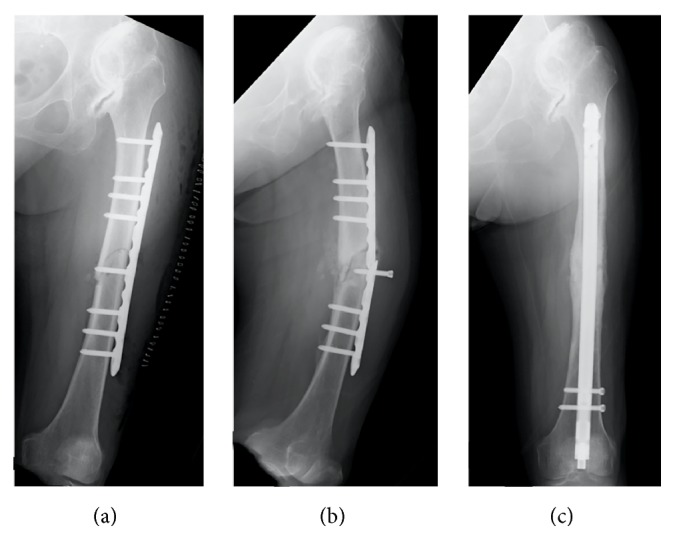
(a) AP image is shown, which was obtained after open reduction and internal fixation with an LCP plate. (b) AP radiographs obtained 22 weeks after fracture surgery show plate breakage. (c) AP image obtained 43 weeks after initial fracture surgery; reoperation was performed with retrograde intramedullary nailing.
